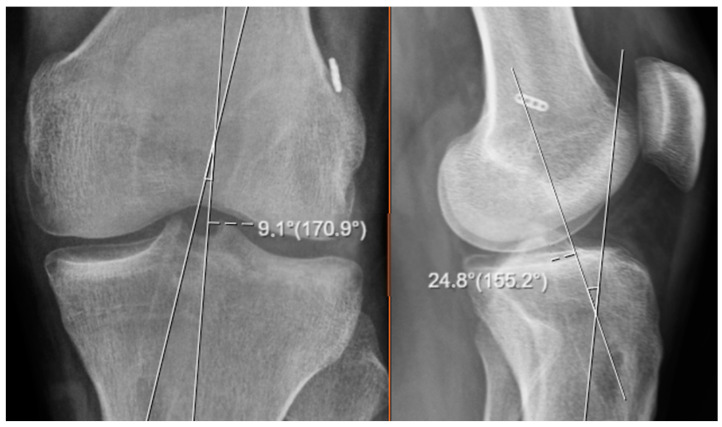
Image showing the measurement of tunnel angles on knee roentgenograms.
